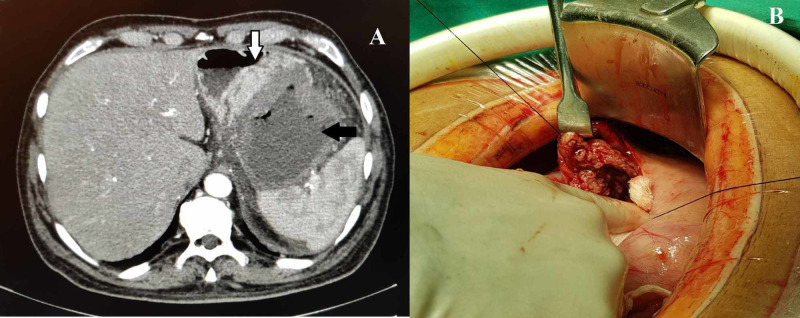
(A) Contrast-enhanced computed tomography of the abdomen showing the walled-off pancreatic necrosis with air foci within it (black arrow) and the hypervascular lesion in the gastric wall (white arrow). (B) Intraoperative photograph showing the gastric submucosal lesion (GIST).
